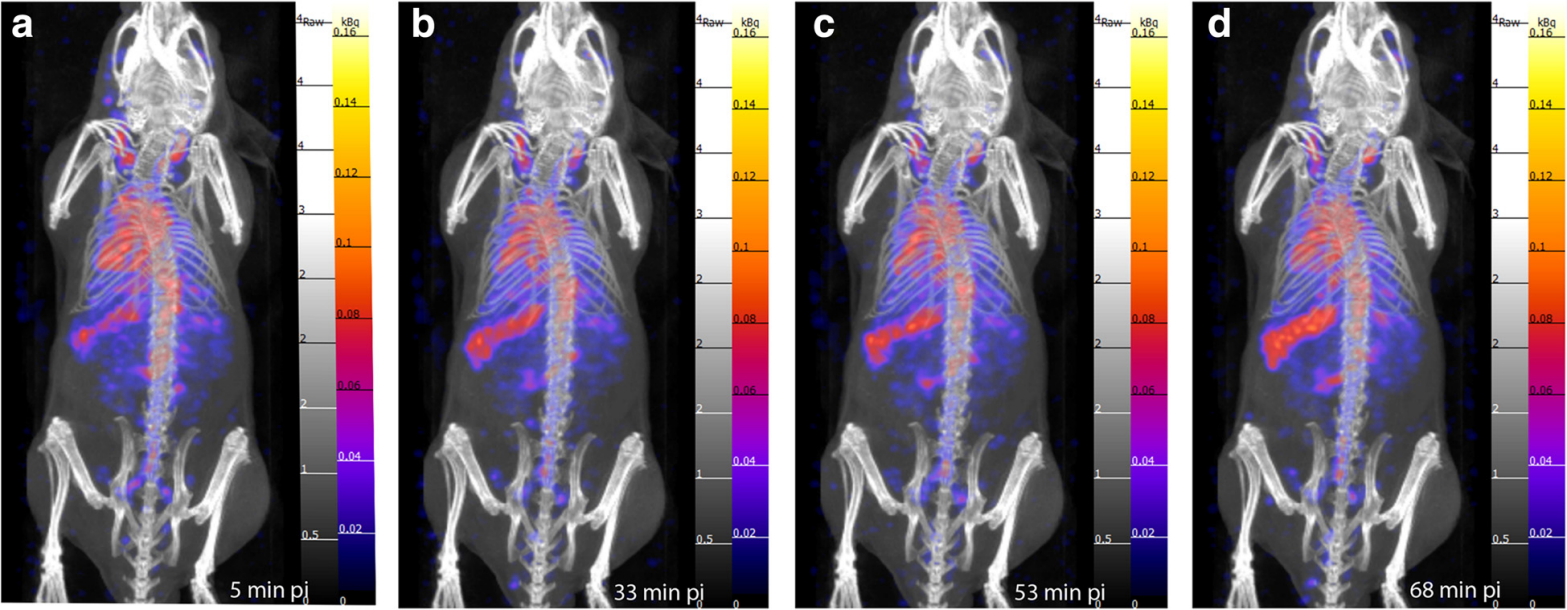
Dynamic SPECT/CT scans. Obtained after administration of 1 mg of ^111^In-PS-b-PEO micelles containing 22 MBq. a 5 min pi. b 33 min pi. c 53 min pi. d 68 min pi
